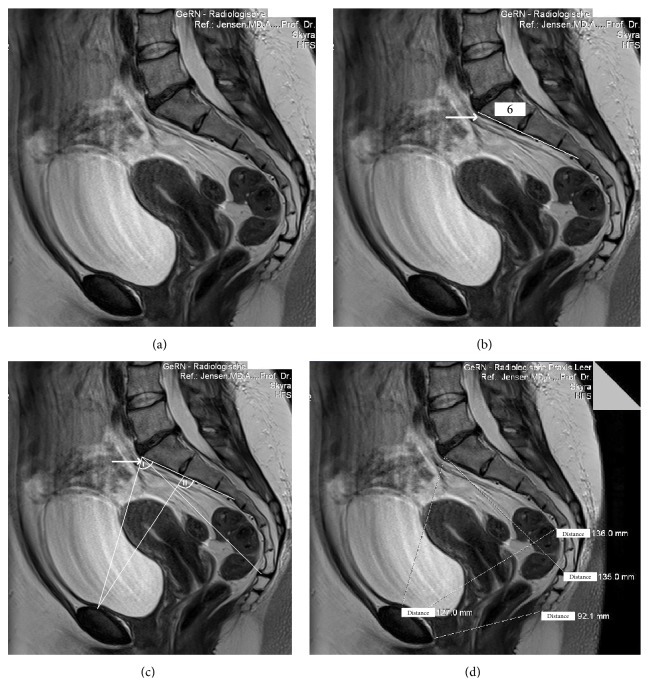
MRI of the maternal pelvis (I.H.). (a) This MRI documents a “long pelvis” [2] with assimilated (fused) lumbar vertebral body to form a sacral bone with an excessive vertebral body (arrow). (b) The “long pelvis” has 6 vertebral bones instead of the normal 5. In addition, the 6 sacral bones in the “long pelvis” fail to be concave; rather they form a straight plane (indicated by the straight line), which reduces the capacity of the mid-pelvis. (c) The pelvic inlet angle is < 90° (angle I) in a “long pelvis” [2]. (d) The distance from the top of the 6th (excessive) vertebral body to the end of the sacral bone in this “long pelvis” [2] is prolonged ((d), distance 135 mm) (3-Tesla, T1w TSE triplanar sequence).
